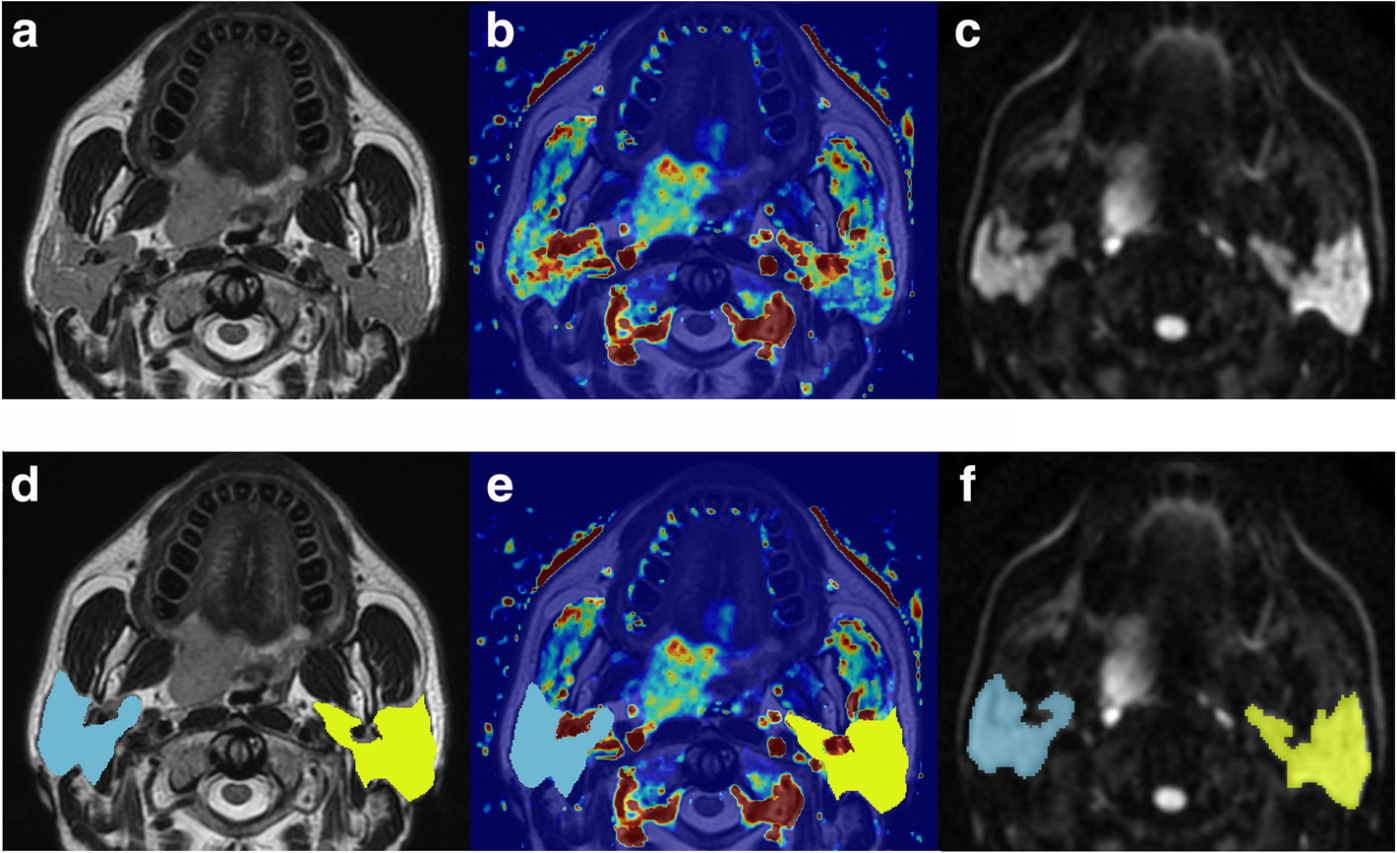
Central section of parotids in a 44-year-old man affected by a tonsil carcinoma on the axial T2-weighted image (a), on the map of K^trans^ (b) and on the diffusion-weighted image obtained with b of 800 s/mm^2^ (c) in the same anatomic location. Bottom: user-defined parotid contours overlaid on the axial T2-weighted image (d) and on the corresponding map of K^trans^ (e) and diffusion-weighted image obtained with b of 800 s/mm^2^ (f)
